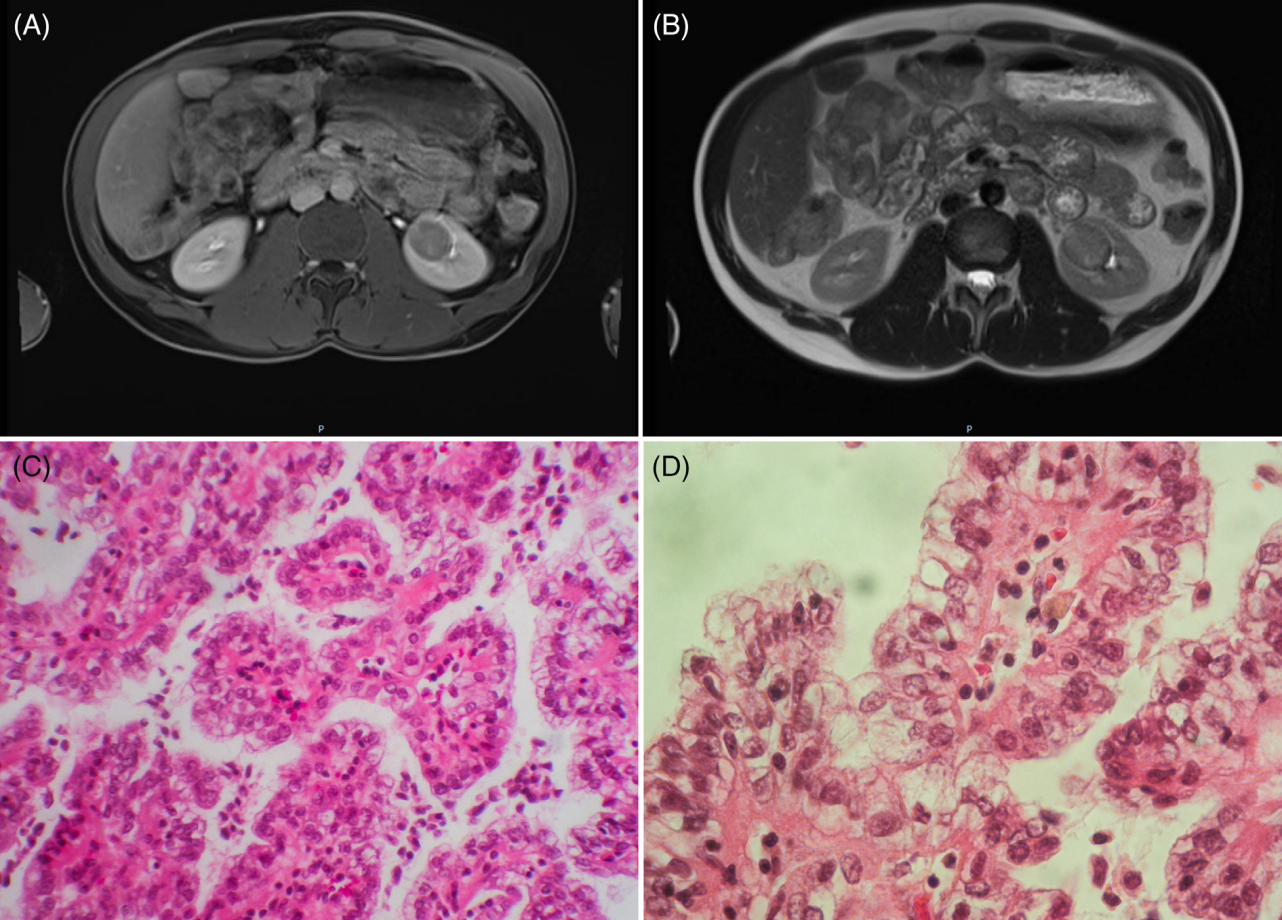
A, Renal MRI, T1 weighted image, with injection. Nodular lesion, isointense. B, Renal MRI, T2 weighted image, with injection. Nodular lesion, discreetly hyperintense with discreet contrast enhancement after injection. C, Papillary renal cell carcinoma type 2, HE. D, Papillary renal cell carcinoma type 2, HE
